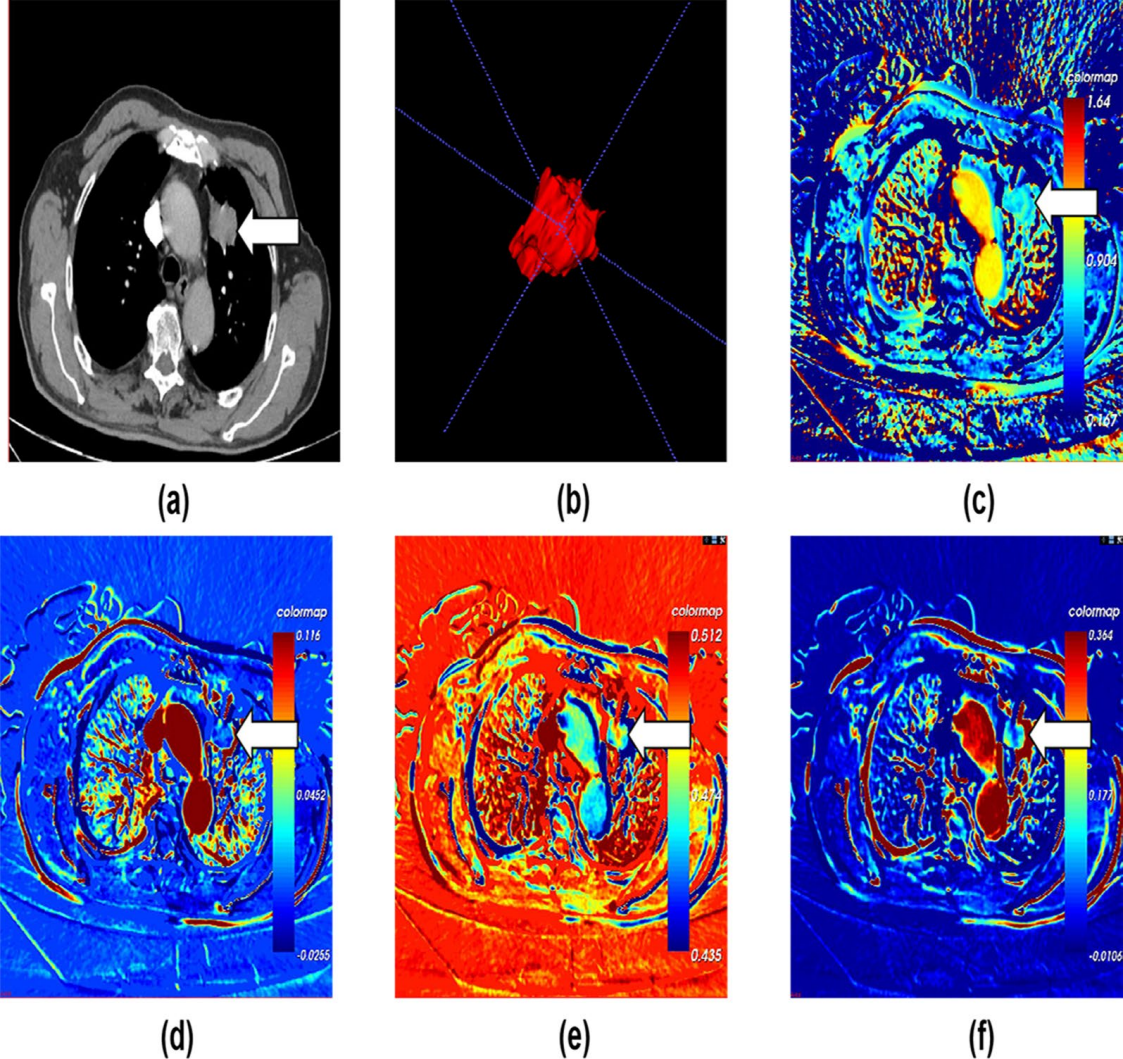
Adenocarcinoma in an 80-year-old man. a The mediastinal window with dynamic three stage enhanced CT scan showing a nodule in left upper lobe, the area indicated by the arrow is the tumour. b 3D ROI of primary tumor lesion. c–f Perfusion maps of pulmonary artery, bronchial artery, perfusion index and arterial enhancement fraction. Arrows indicate the tumours
